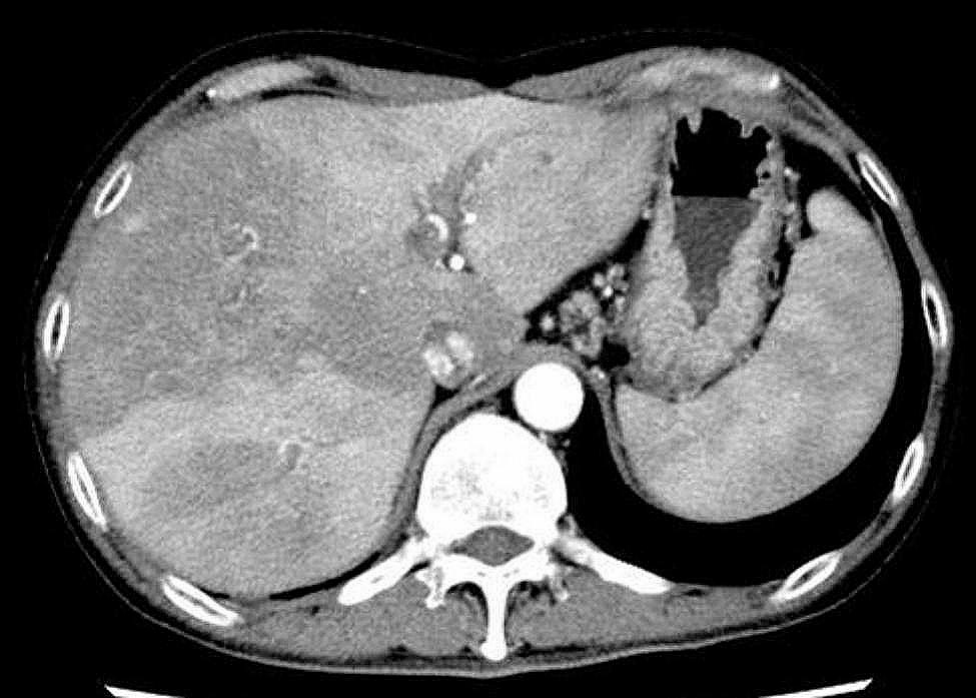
Computed tomography showing early inhomogeneous enhancement of the liver
